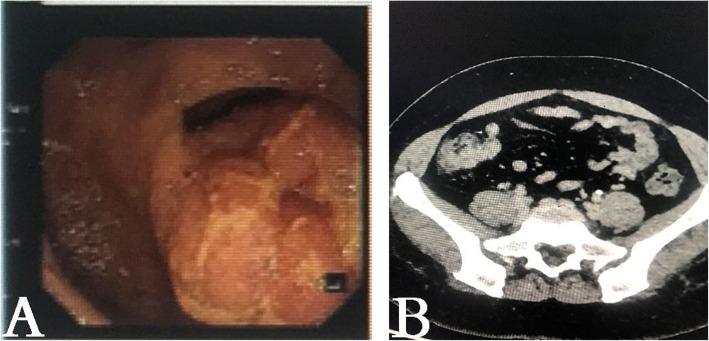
a Colonoscopy shows a protuberant mass, size 2.5 cm, in the caecum. b Abdomen CT scan: the delayed phase of contrast-enhanced CT showed localized intestinal wall thickness in the caecum and unclear serosa layer. No lymph nodes were noted
